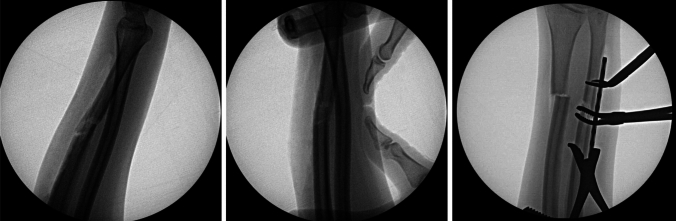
Intraoperative radiographs demonstrating plastic deformation of the radius, unsuccessful closed osteoclasis, and radial shaft osteotomy
